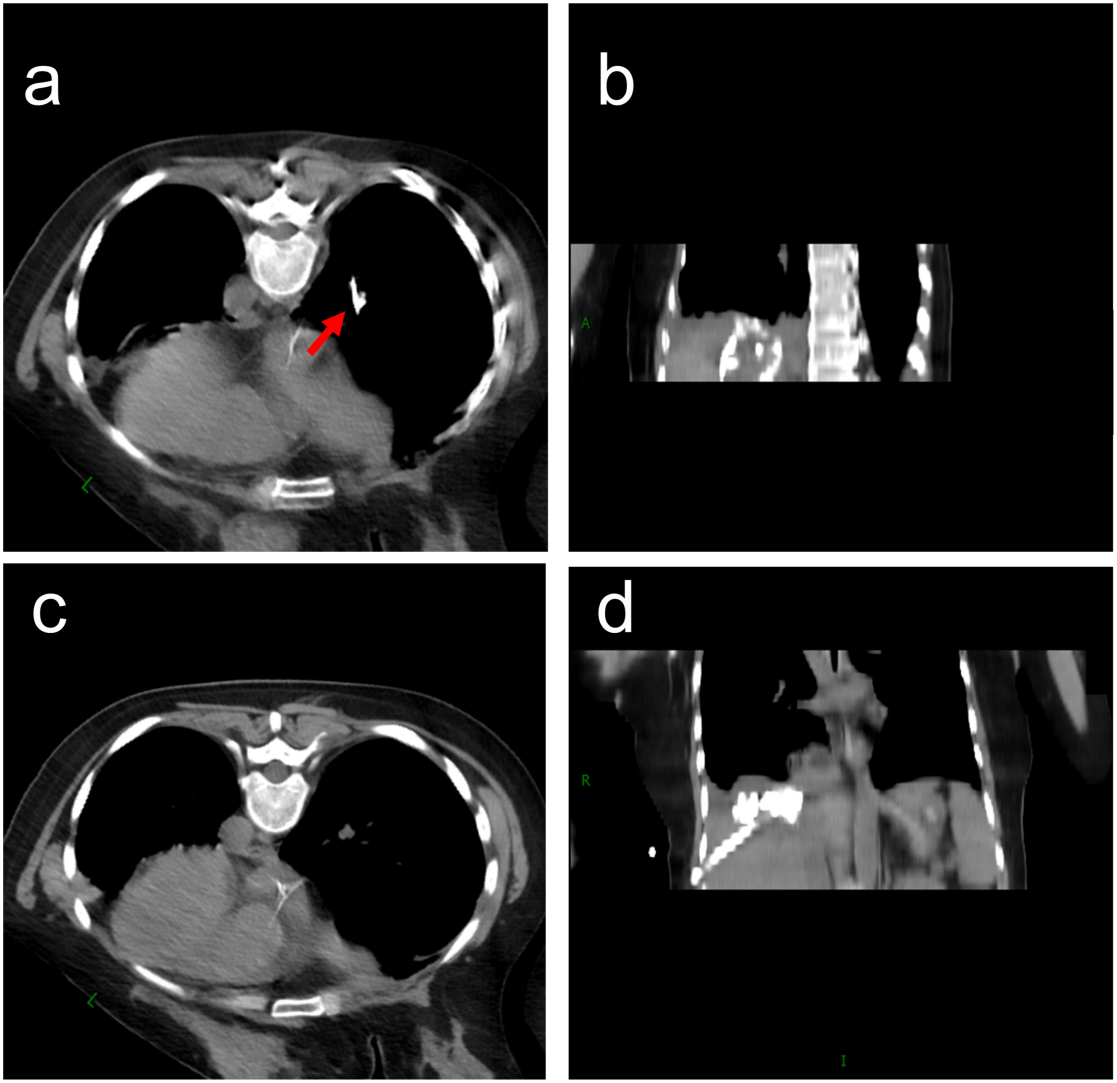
Computed tomography (CT) images of percutaneous microwave ablation (PMWA) for bronchobiliary fistula (BBF). (a) PMWA procedure on the fistula tract on October 4, 2022 (red arrow indicates the ablation needle adjacent to the contrast-filled fistula); (b) coronal CT image of the fistula after PMWA on October 4, 2022; (c) axial CT image of the fistula after PMWA on October 4, 2022; (d) repeated coronal CT image of the fistula on October 12, 2022.
